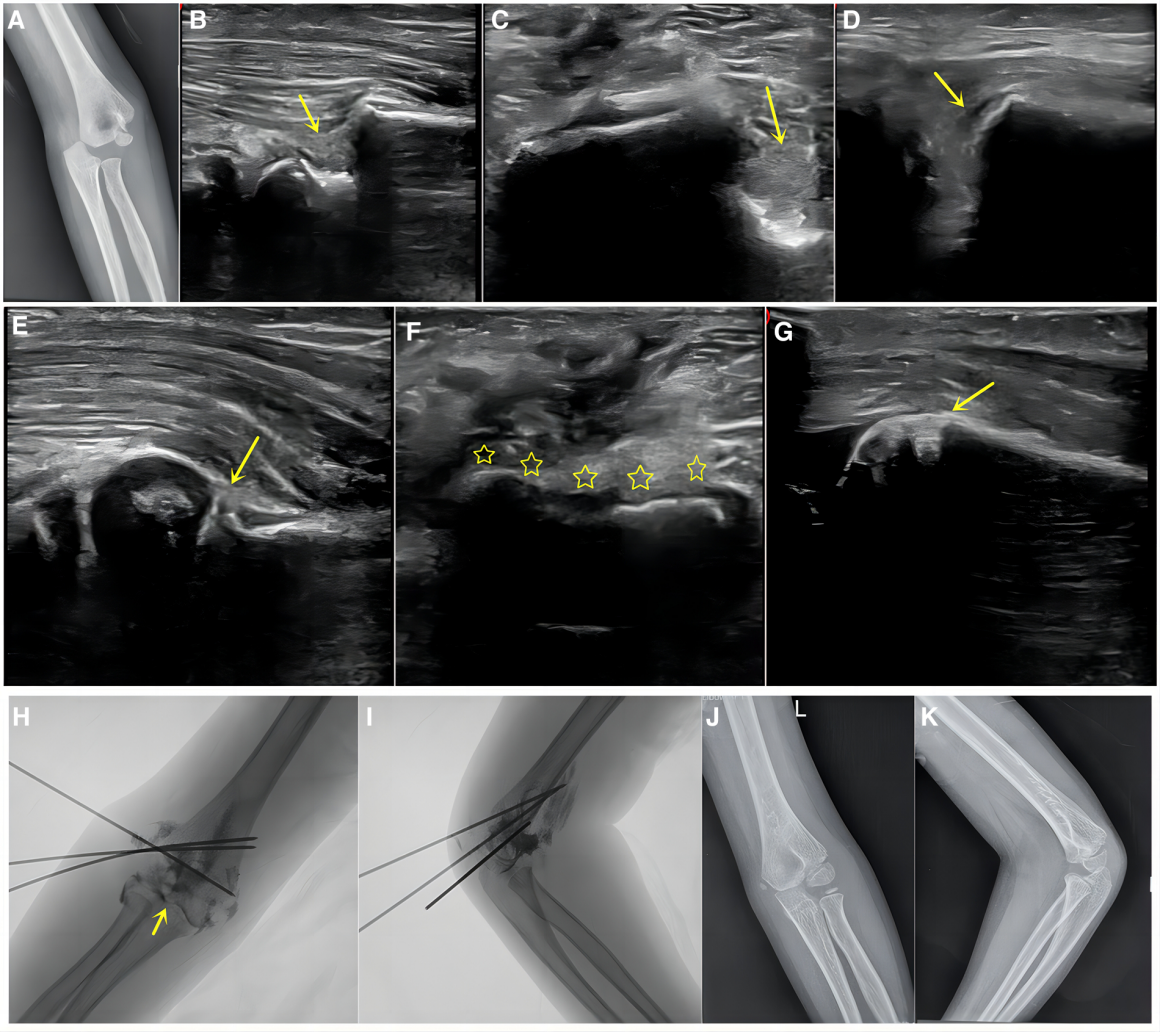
Boy, 6 years old, left humeral lateral condyle fracture, dislocation type. (A) Preoperative x-ray revealed dislocation of the elbow joint with a rotated fragment. (B–D) Before reduction, severe displacement can be seen on ultrasound in the sagittal, coronal, and posterior lateral metaphyseal directions (arrow). (E–G) After reduction and fixation, good reduction can be seen in the sagittal, coronal, and posterior lateral metaphyseal directions on ultrasound, with continuous cartilage hinge and normal condylar-shaft angle. (H,I) Postoperative imaging showed continuous cartilage hinge after reduction (arrow), divergent Kirschner wires fixation. (J,K) After 21 months of postoperative follow-up, it was found that the fracture was normal, without lateral spur and the function was excellent.
